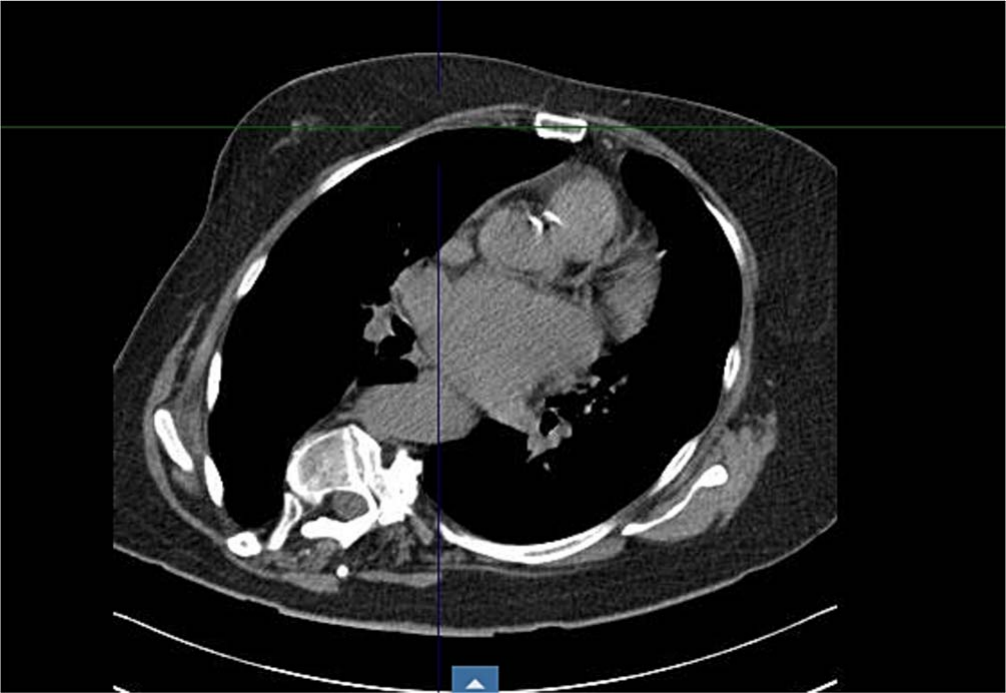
Axial CT at level of 2nd ICS.
